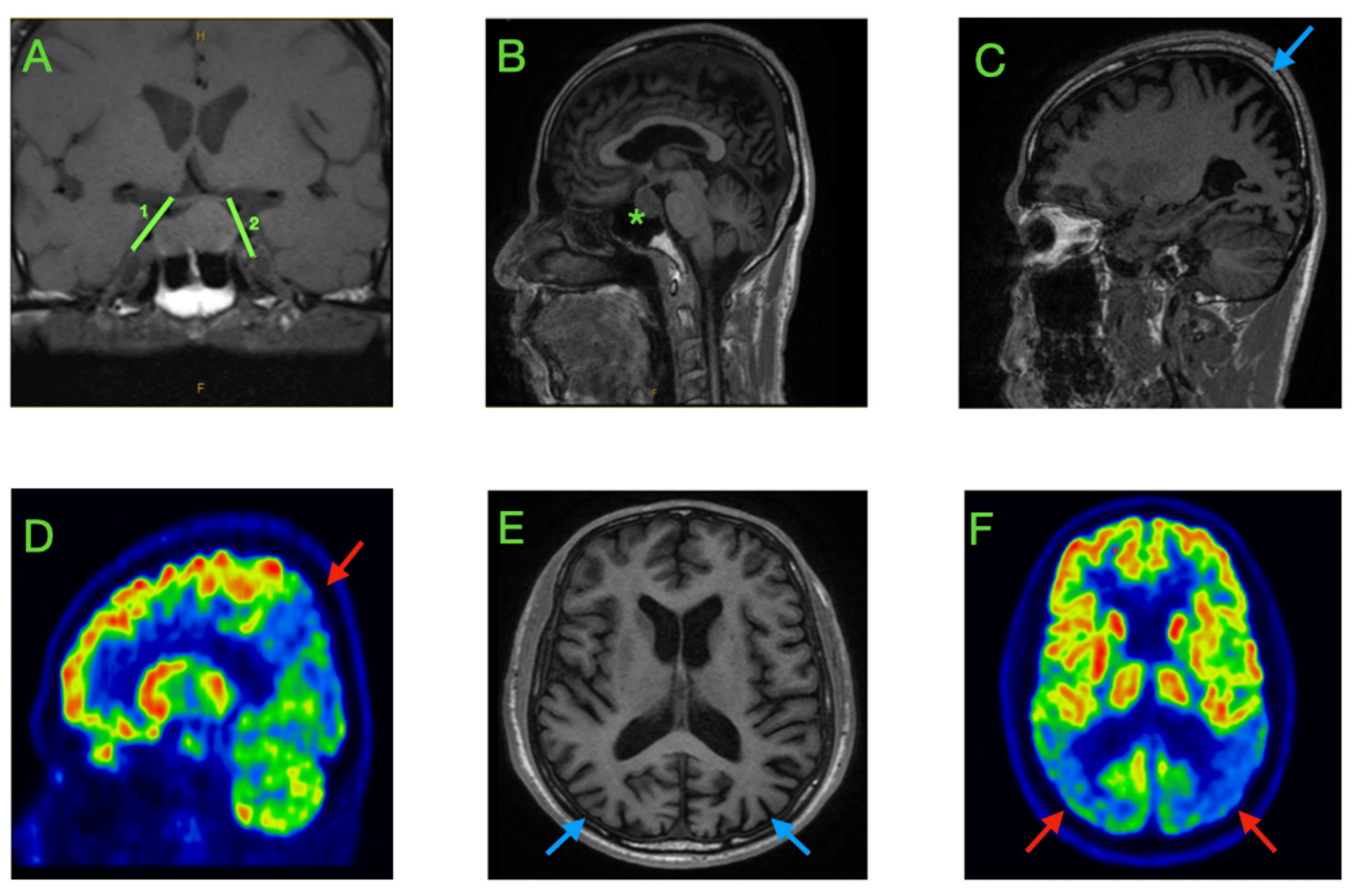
Magnetic resonance imaging and 18F-fluorodeoxyglucose positron emission tomography demonstrating pituitary macroadenoma and parieto-occipital changes. Coronal (A) and sagittal (B) T1-weighted magnetic resonance imaging (MRI) views demonstrate a pituitary macroadenoma (asterisk) measuring 14 x 17 mm (dimensions indicated in green). Sagittal MRI (C) demonstrates parietal cortical atrophy (blue arrow) with corresponding parieto-occipital hypometabolism on 18F-fluorodeoxyglucose positron emission tomography (FDG-PET) (D, red arrow). Transverse MRI (E) and FDG-PET (F) views similarly demonstrate parietal atrophy (blue arrows) and hypometabolism (red arrows). The posterior cortical changes support the diagnosis of posterior cortical atrophy, while the stable macroadenoma accounts for the right homonymous hemianopia.
